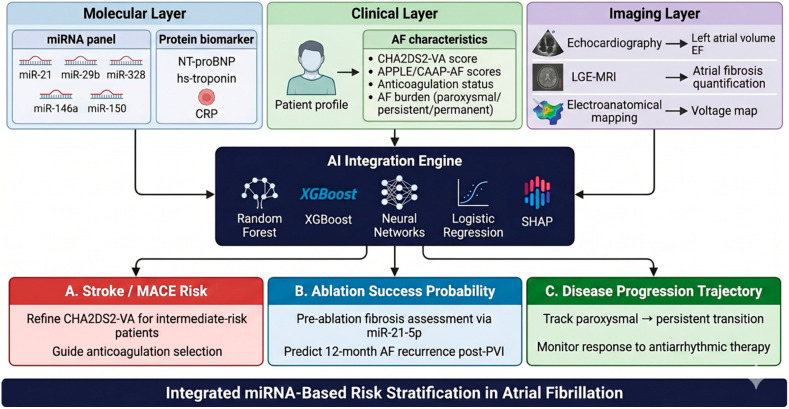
Proposed conceptual framework for integrated miRNA-based risk stratification in atrial fibrillation. Three input data layers (molecular: miRNA panel + protein biomarkers; clinical: patient profile + AF characteristics; Imaging: echocardiography + LGE-MRI + electroanatomical mapping) are integrated by AI-driven machine learning algorithms to generate individualized scores for three clinical decision domains: (A) stroke/MACE risk stratification; (B) ablation success probability; (C) disease progression trajectory. AF: atrial fibrillation; CHA2DS2-VA: stroke risk score (2024 ESC); LGE-MRI: late gadolinium enhancement MRI; LSTM: long short-term memory network; MACE: major adverse cardiovascular events; NT-proBNP: N-terminal pro-brain natriuretic peptide; PVI: pulmonary vein isolation; SHaAP: SHapley Additive exPlanations; SNP: single nucleotide polymorphism.
